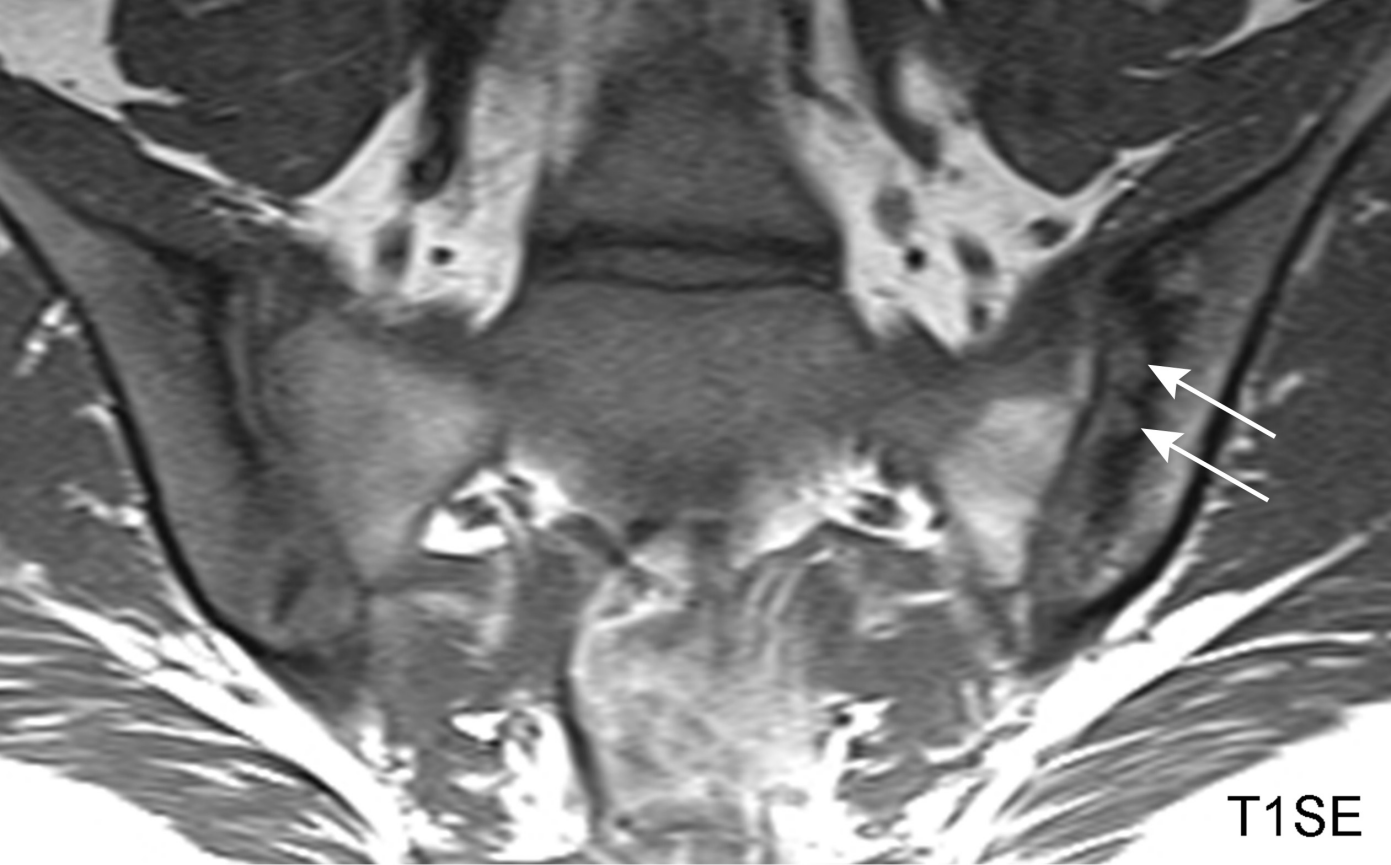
Classical erosion (T1SE sequence). HLA B27 positive, 18-year-old female AS patient with inflammatory back pain for two years. Erosion in the left ilium (arrows) is characterized by loss of visualization of the cortical bone. The erosions are well visualized because they are surrounded by sclerosis as evidenced by very dark signal in the adjacent bone marrow. HLA-B27, human leucocyte antigen B27; T1SE, T1-weighted spin echo sequence.
